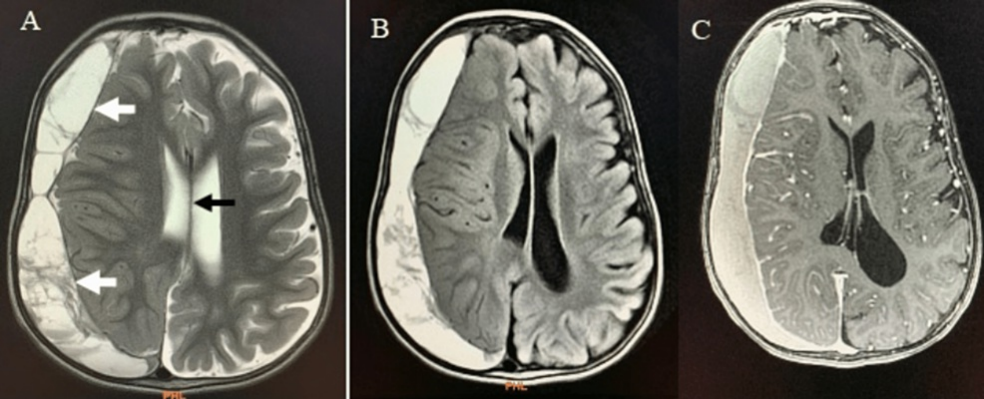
(A) Axial section of brain MRI T2 showing large subdural hematoma (white arrows) in right-sided frontal and temporal lobes with marked positive mass effect and midline shift (black arrow) (B) Axial section of brain MRI FLAIR and (C) Axial section of brain MRI contrast-enhanced.
